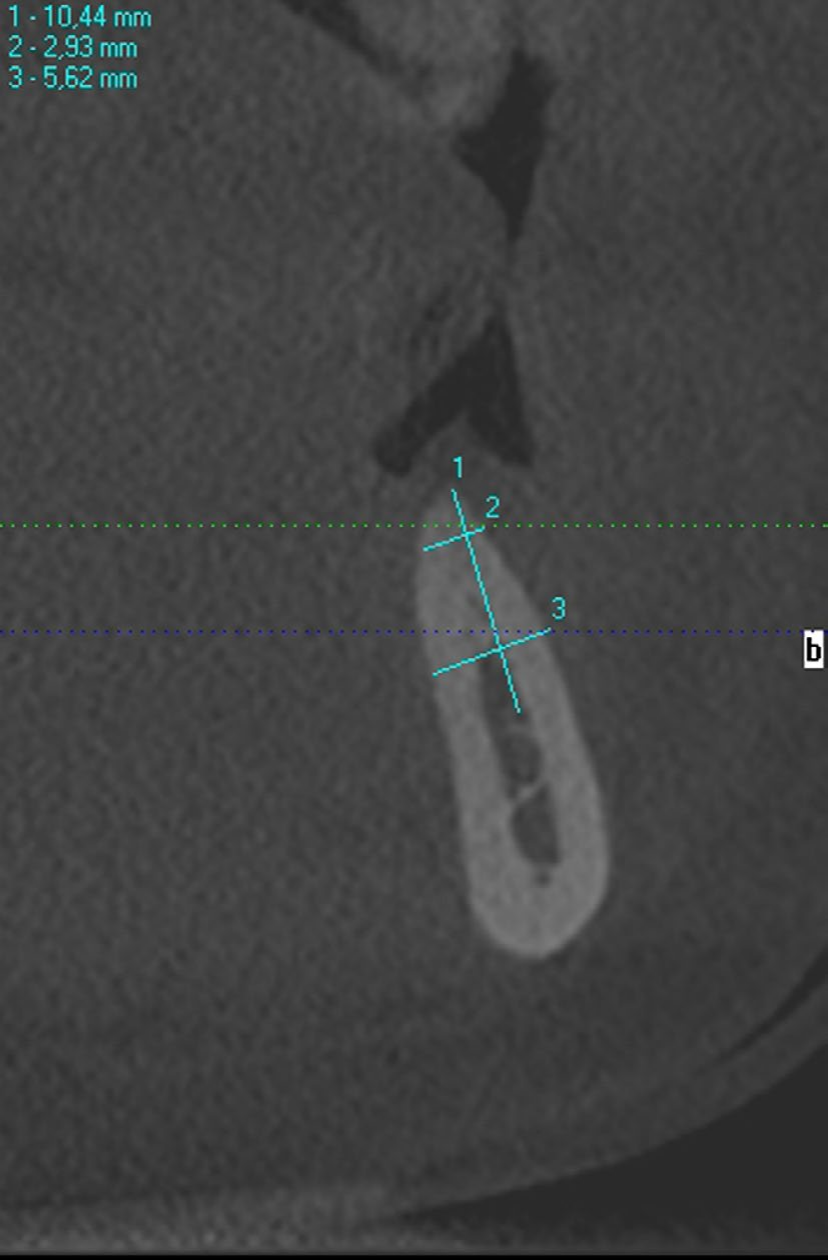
Initial condition on CBCT
